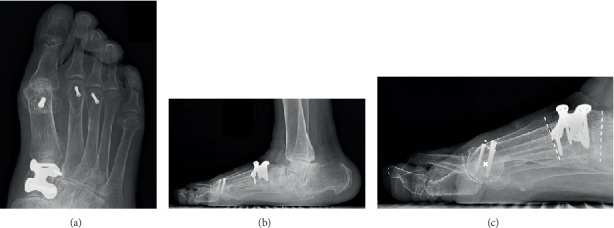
Postoperative weight-bearing radiographs of the foot. (a) Anteroposterior view of the foot showing improved hallux valgus deformity and congruent metatarsophalangeal joint. (b, c) Lateral view of the foot showing improvement of the elevated first metatarsal, plantar deviation of the proximal phalanx, and interphalangeal hyperextension. The two arrowheads indicate the distance between two dorsal cortices of the first and second metatarsals, indicating improvement of the elevated first metatarsal compared with Figure 2(c). The cross indicates the approximate center of the metatarsal head. Dotted lines drawn along the naviculocuneiform and tarsometatarsal joints demonstrate the improvement of plantar flexion by approximately 10° after the Cotton osteotomy.
